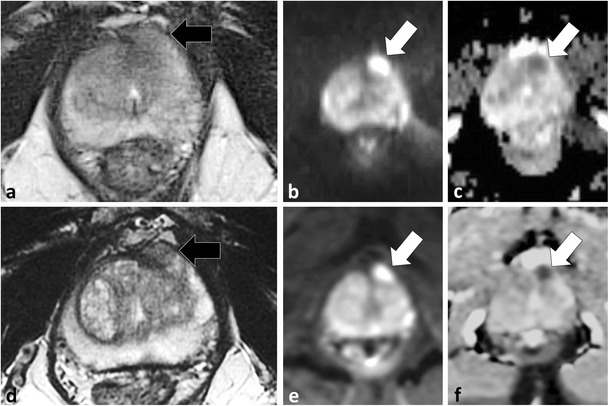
A 63-year-old patient with elevated PSA and previously negative non-targeted TRUS-guided biopsy with persistent clinical suspicion of prostate cancer underwent MP-MRI in two separate sessions within 3 months demonstrating the loss of contrast with BLADE/PROPELLER imaging. Axial T2W BLADE a, b1000 mm²/s EPI b and ADC map c demonstrate a suspicious focus of restricted diffusion in the left middle anterior horn of the PZ (*white arrows*), which is not visible on axial T2W BLADE (*black arrow* in a). Repeat examination performed using T2W FSE (d) reveals a T2 hypointense nodule in the same location (*black arrow* in d) with persistent restricted diffusion (*white arrows* in e and f)
